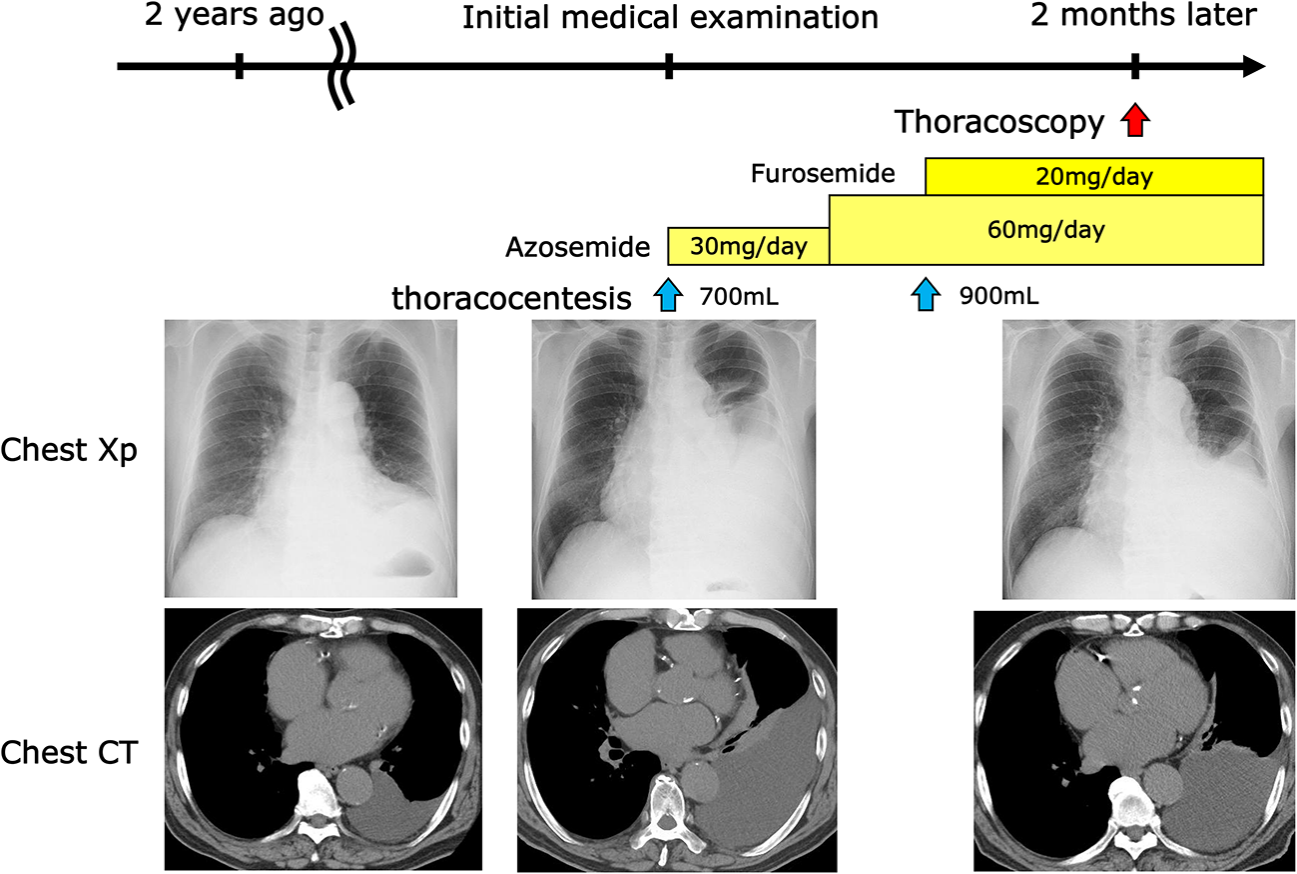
Left pleural effusion was noted on computed tomography (CT) scan approximately 2 years ago, but it was of a small volume and was under observation. A chest x‐ray revealed a significant increase in left pleural fluid, leading to a referral to our department. Although oral diuretics were initiated for treatment, but the left pleural effusion did not diminish. Subsequently, we conducted thoracoscopy for a definitive diagnosis.
